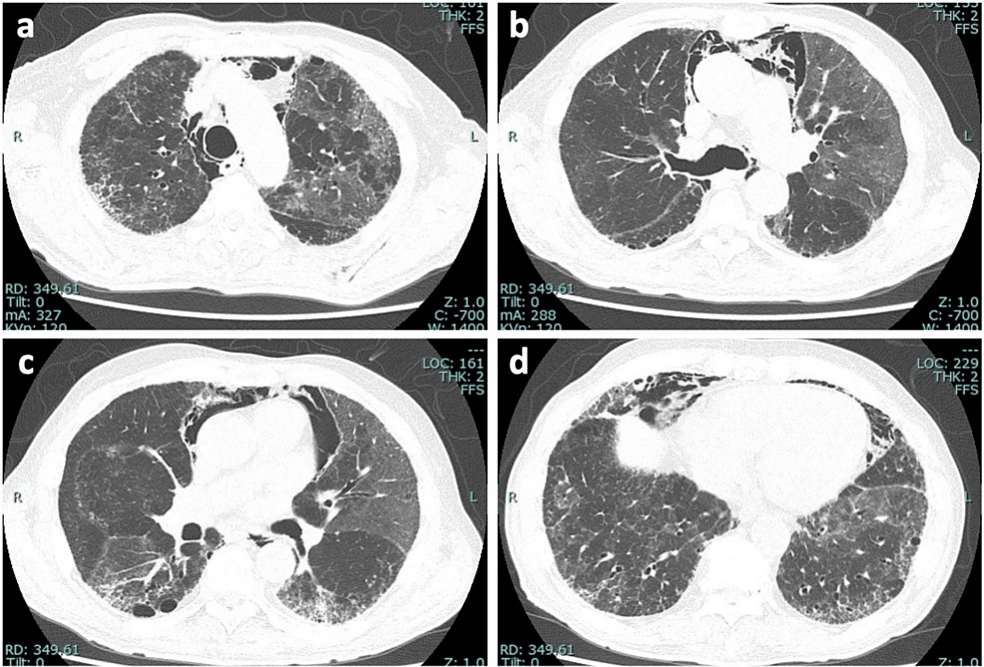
Following chest CT images of the patient on day 44. (a-d) The sequential panels from a high-resolution chest CT demonstrate marked improvements in ground-glass opacities and consolidations. Persistent honeycombing-like cysts and reticular patterns are visible, predominantly within the basal segments of the lungs. Mediastinal emphysema is also evident.
